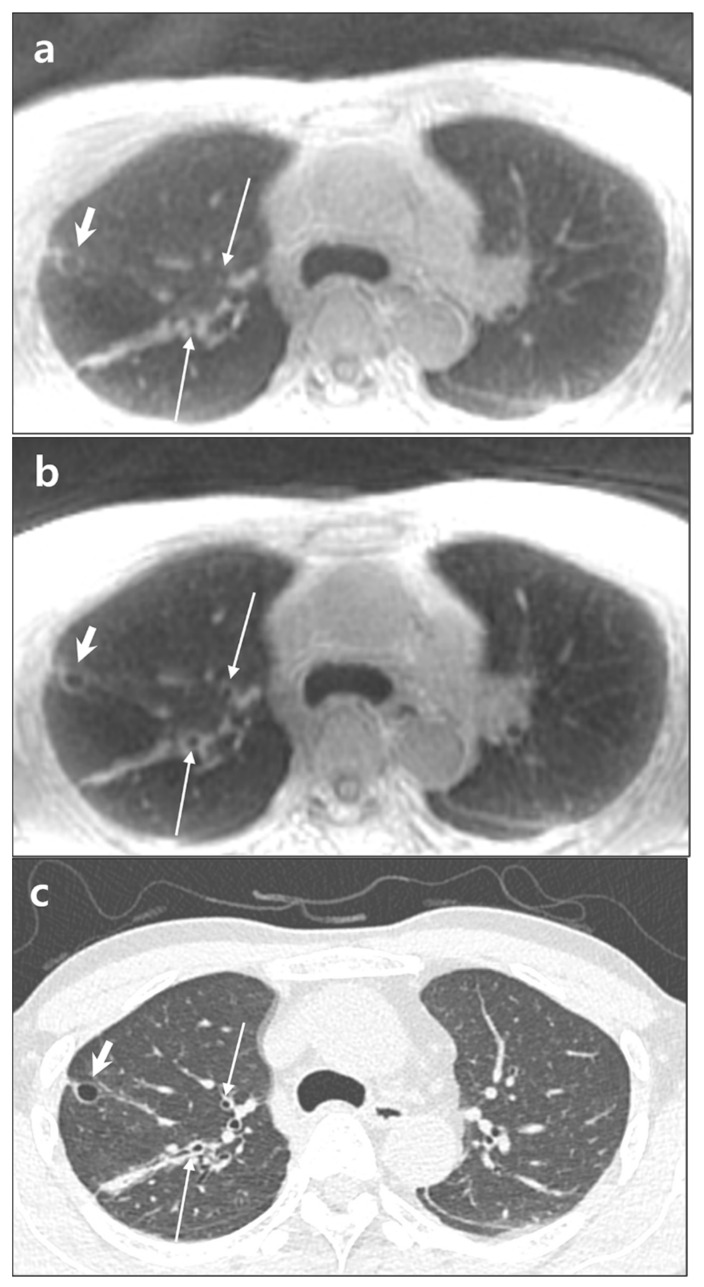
A 55-year-old male with lung cyst in the right upper lobe. Note signal difference between lung parenchyma and cyst (short arrows) and visualization of peripheral bronchi (thin arrows) in ZTE-CAA (a) and ZTE-AIR (b). Chest CT image (c) is presented as reference. ZTE-CAA, zero echo time lung MR image using conventional anterior array coil; ZTE-AIR, zero echo time lung MR image using adaptive image receive coil.
